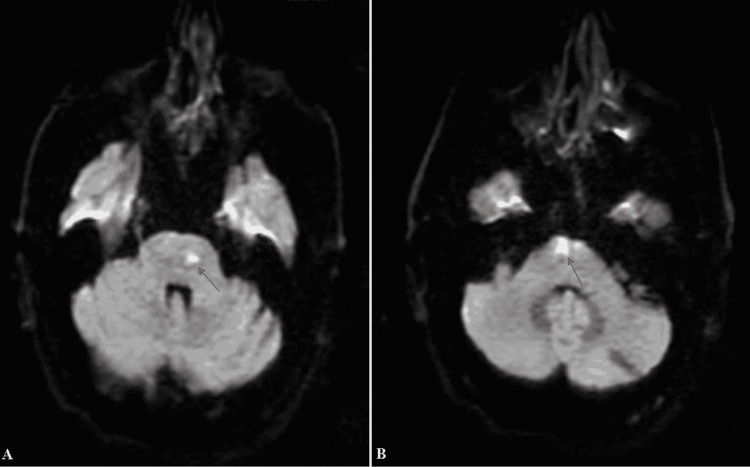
Axial diffusion brain MRI images Axial diffusion brain MRI shows an ischemic stroke in the pons (A) and in the anterior bulbar area (B)
